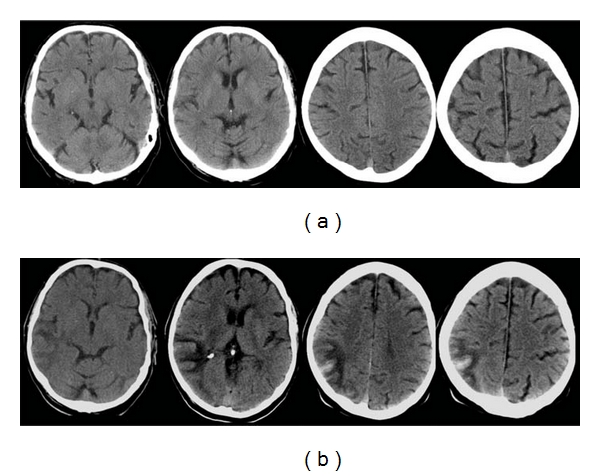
83-year-old man with atrial fibrillation, he presented with left-sided weakness and neglect. (a) Axial unenhanced CT images within 3 hours of symptom onset show no acute changes. (b) Axial unenhanced CT images 10 days after stroke show a hemorrhage within a wedge-shaped infarct in the right posterior parietal lobe. The surrounding hypodense area follows the topography of the middle cerebral artery, reaching out to the cortex and superiorly, consistent with a hemorrhagic infarct. Stage HI c2 [1].
